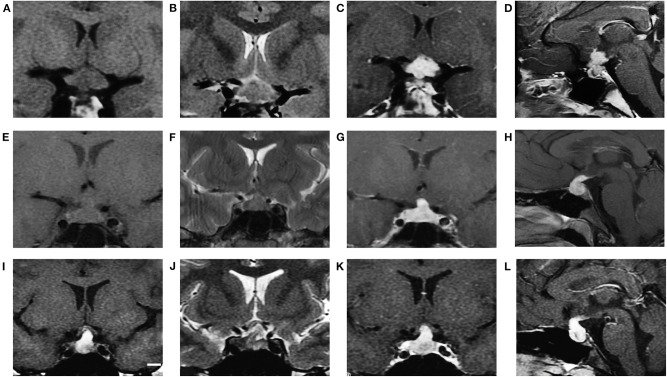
MRI characteristics of GCT, LCH and CP (cases 7, 46, and 53, respectively). GCT showed isointensity on T1WI (A coronal), iso to hyperintensity on T2WI (B coronal) and obvious enhancement after gadolinium injection (C coronal, D sagittal). LCH showed isointensity on T1WI (E coronal), isointensity on T2WI (F coronal) and obvious enhancement after gadolinium injection (G coronal, H sagittal). CP showed hyperintensity on T1WI (I coronal), hyperintensity on T2WI (J coronal) and obvious heterogeneous enhancement (K coronal, L sagittal).
